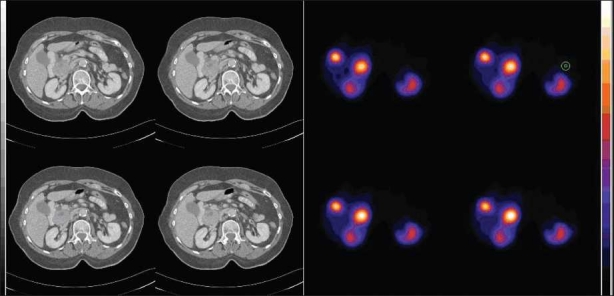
An example of image fusion where uptake of activity in SPECT image (right) can be localized to the pancreatic head (centre uptake) and physiological uptake in gall bladder (upper left uptake) and kidneys (inferior uptakes)
